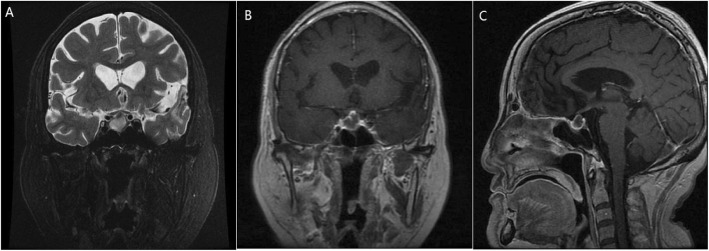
Pituitary MRI at admission showed an enlarged pituitary gland with heterogenous high signal on coronal T2 weighted image (Fig. 2a) and central low signal with peripheral rim enhancement on post contrast T1 weighted coronal (Fig. 2b) and sagittal (Fig. 2c) images causing mild compression on the optic chiasm, which is suggestive of acute pituitary apoplexy
